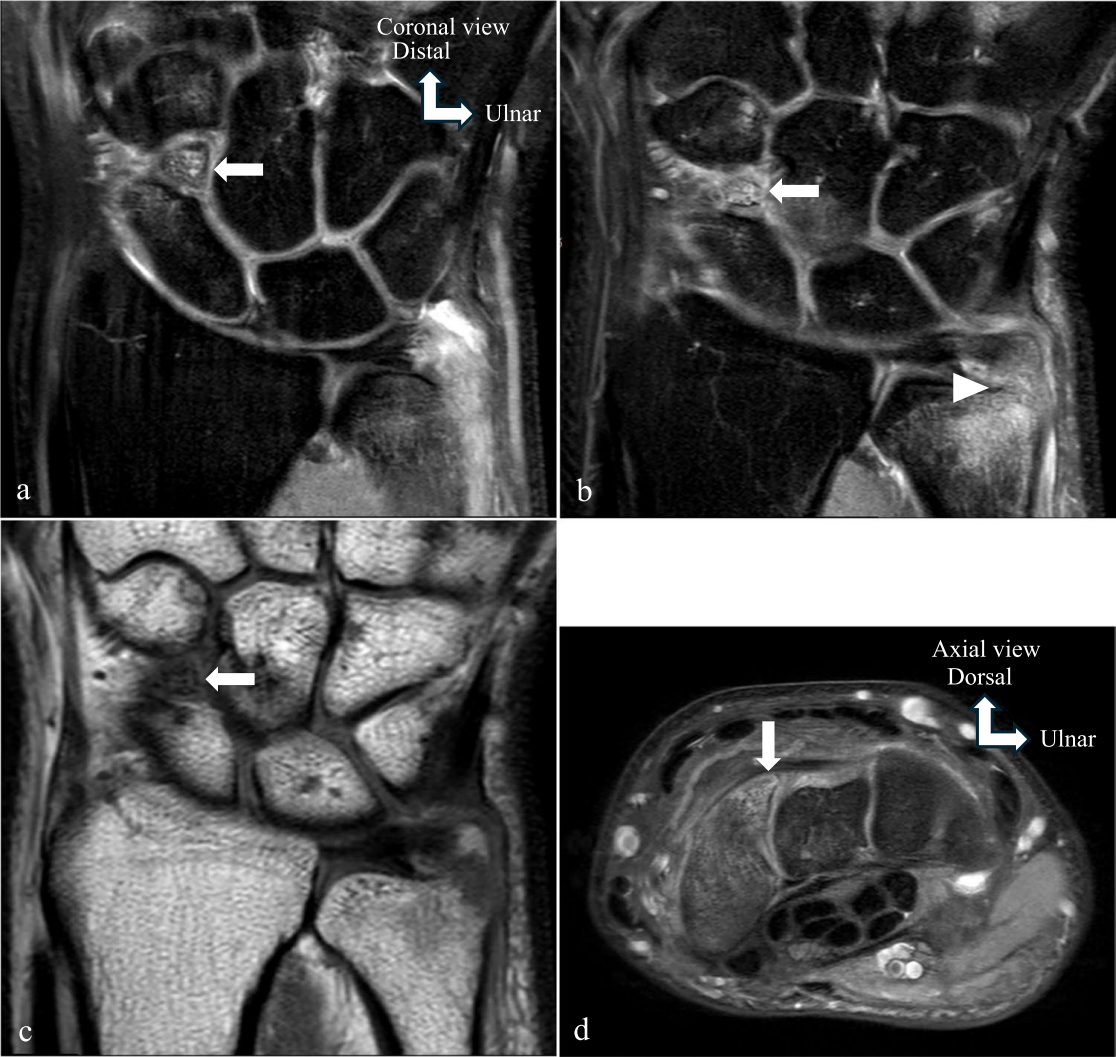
Os centrale carpi bone bruising. a, b Coronal MRI in PD FS. c Coronal MRI in T1-weighted TSE. d Axial MRI in PD FS. MRI was performed to better characterize the presumed scaphoid fracture. The MRI images confirmed a fracture of the base of the ulnar styloid (arrowhead), and also showed a triangular ossicle in the dorsal aspect of the articulation between the scaphoid, capitate, and trapezoid bones (arrows). The adjacent distal pole of the scaphoid and this ossicle presented intense edema. The presence of marked smooth sclerotic edges of this ossicle and the adjacent distal pole of the scaphoid, as well as subchondral sclerosis and fibrocartilaginous tissue on the surface between these two osseous components, called into question the diagnosis of a fracture of the distal pole of the scaphoid and confirmed os centrale carpi bone bruising
